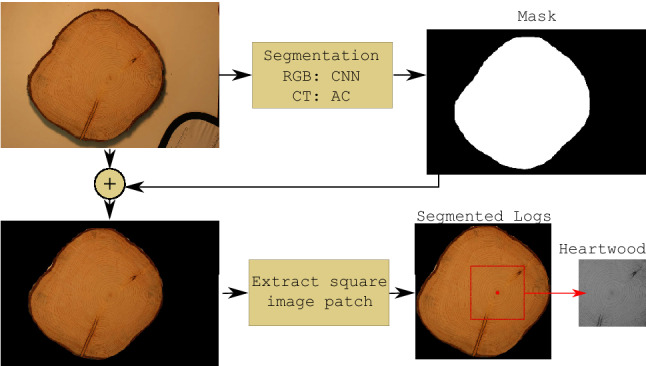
Segmentation using a CNN for RGB images and the Active Contour (AC) method for CT images followed by the patch extraction of the CS-Images
